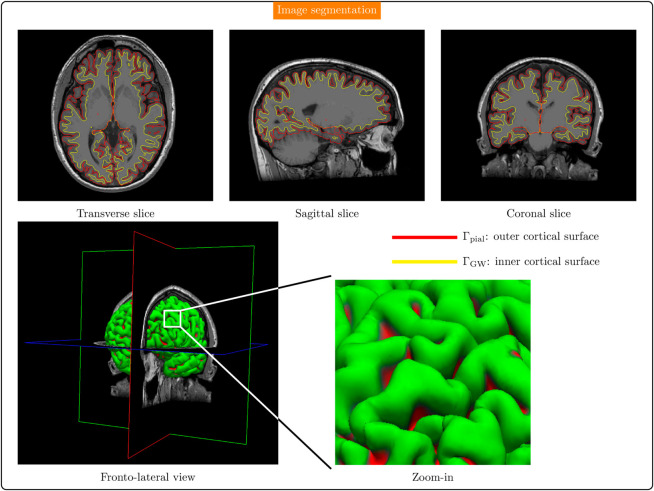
Segmentation of the magnetic resonance image dataset featuring the inner (yellow) and outer (red) cortical surfaces for three orthogonal planes. Sulci and gyri can be appreciated in the zoomed-in area performed over the fronto-lateral view.
